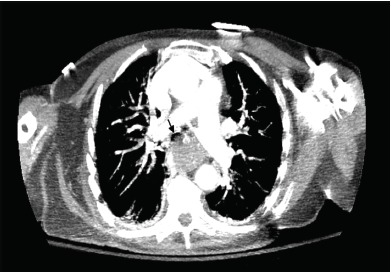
Computed tomography (axial view) demonstrating near obliteration of the trachea at the level of the manubrium secondary to esophageal distention (black arrow).
